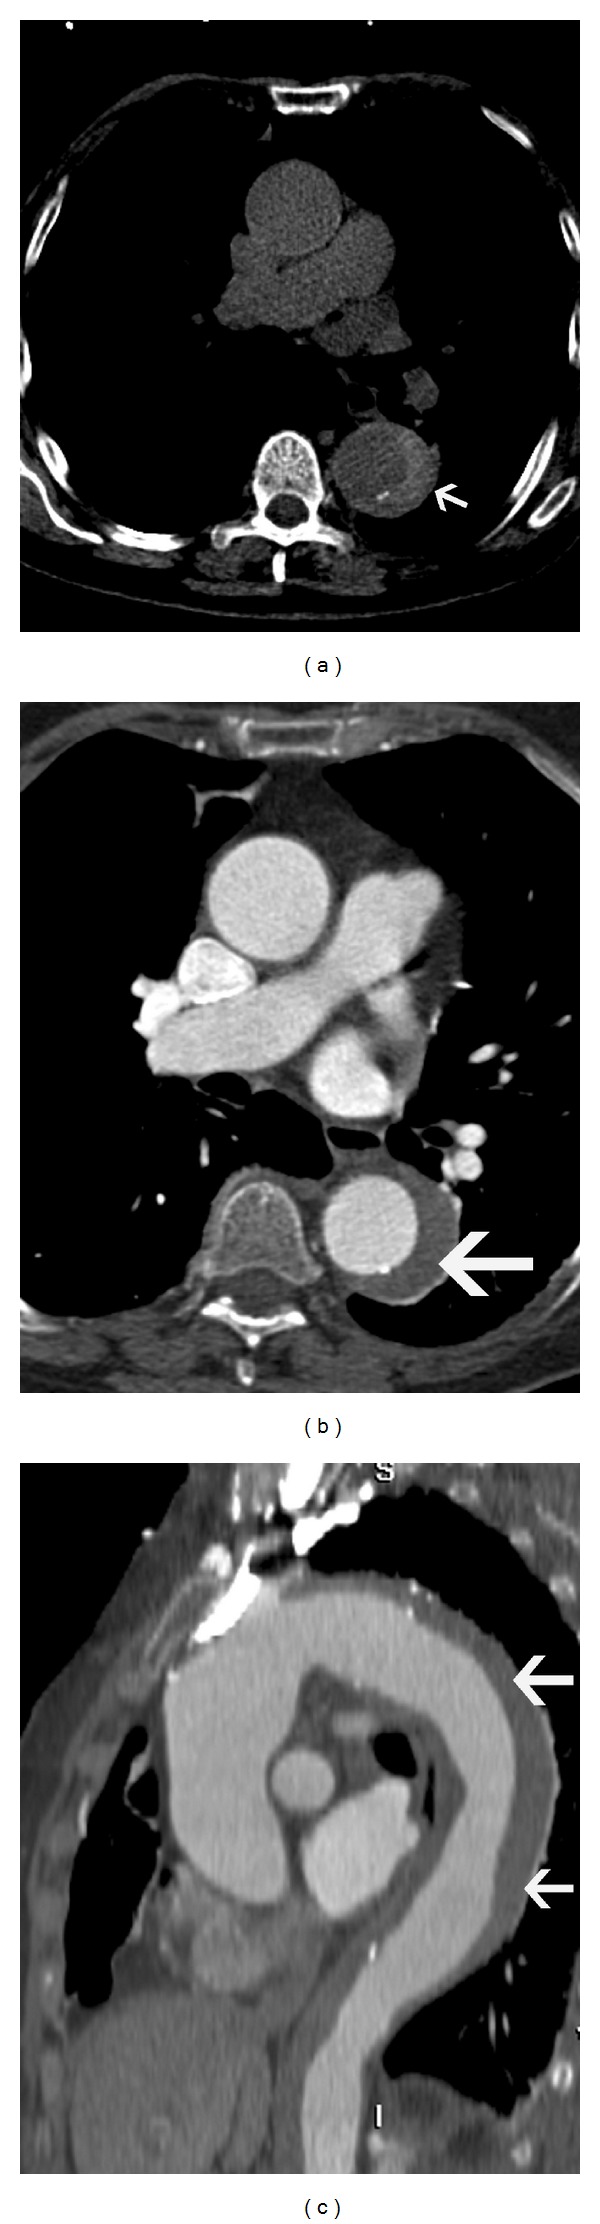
Intramural  hematoma—(a) noncontrast axial CT scan of a 67-year-old male with acute chest pain shows a high density collection (arrow) in the periphery of the descending aorta, which is internally displacing intimal calcification. (b) Axial postcontrast CT scan of the same patient shows the hypodense collection (arrow) in the periphery of the contrast opacified aorta. Although it appear hypodense, the mean attenuation value was 80 HU, which is higher than that for a thrombus. Also, the intimal calcification goes against a luminal thrombus. (c) Sagittal postcontrast CT scan shows the craniocaudal extent of the intramural hematoma (arrows).
